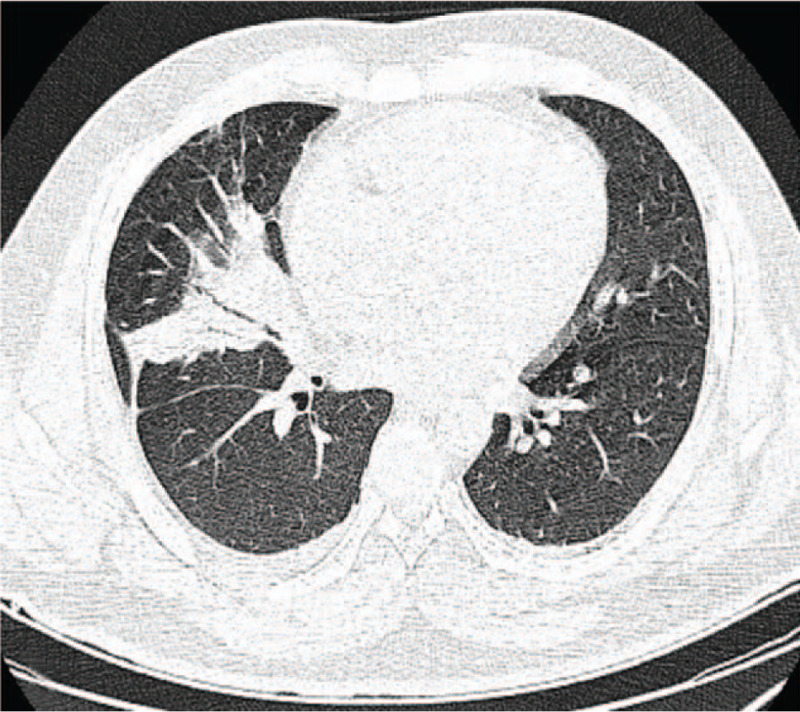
Chest CT on June 13 showed pneumonia.
